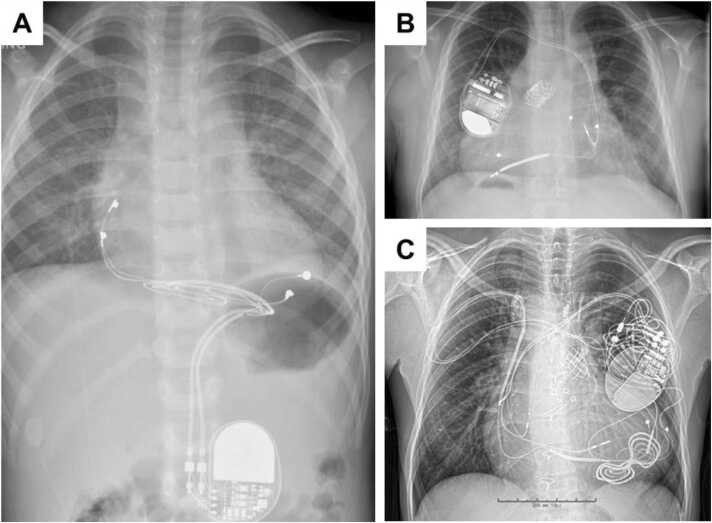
Chest radiographs from patients with congenital heart disease demonstrating a variety of non-MR-conditional CIED systems. (A) A dual chamber epicardial system placed in infancy. (B) A right-sided transvenous system placed in a patient with dextrocardia and a retained epicardial system. (C) A complex hybrid system after many years of CIED management, including bilateral transvenous systems, abandoned leads, an epicardial system tunneled to a subclavian pocket and an epicardial coil. Patients shown in (B) and (C) have an intravascular stent, unrelated to the pacing system.
